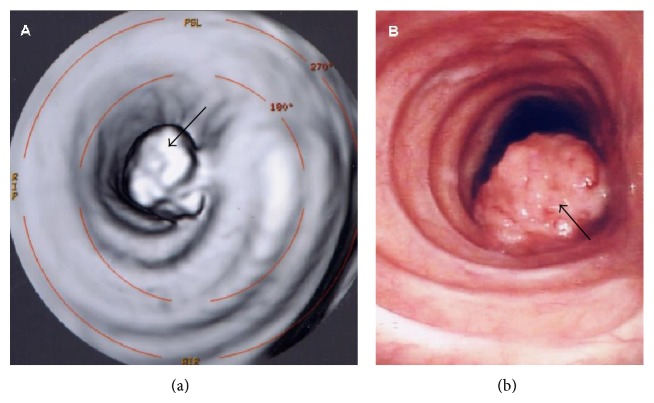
64-year-old female with granular cell tumor of trachea. (a) Virtual bronchoscopy image (16-slice Multidetector CT, GE Medical Systems) and (b) real bronchoscopy image that shows intratracheal sessile lesion (black arrows), characterized by round shape and polylobulated border, originating from left wall of middle portion of trachea and protruding into trachea lumen occupying half of its diameter.
